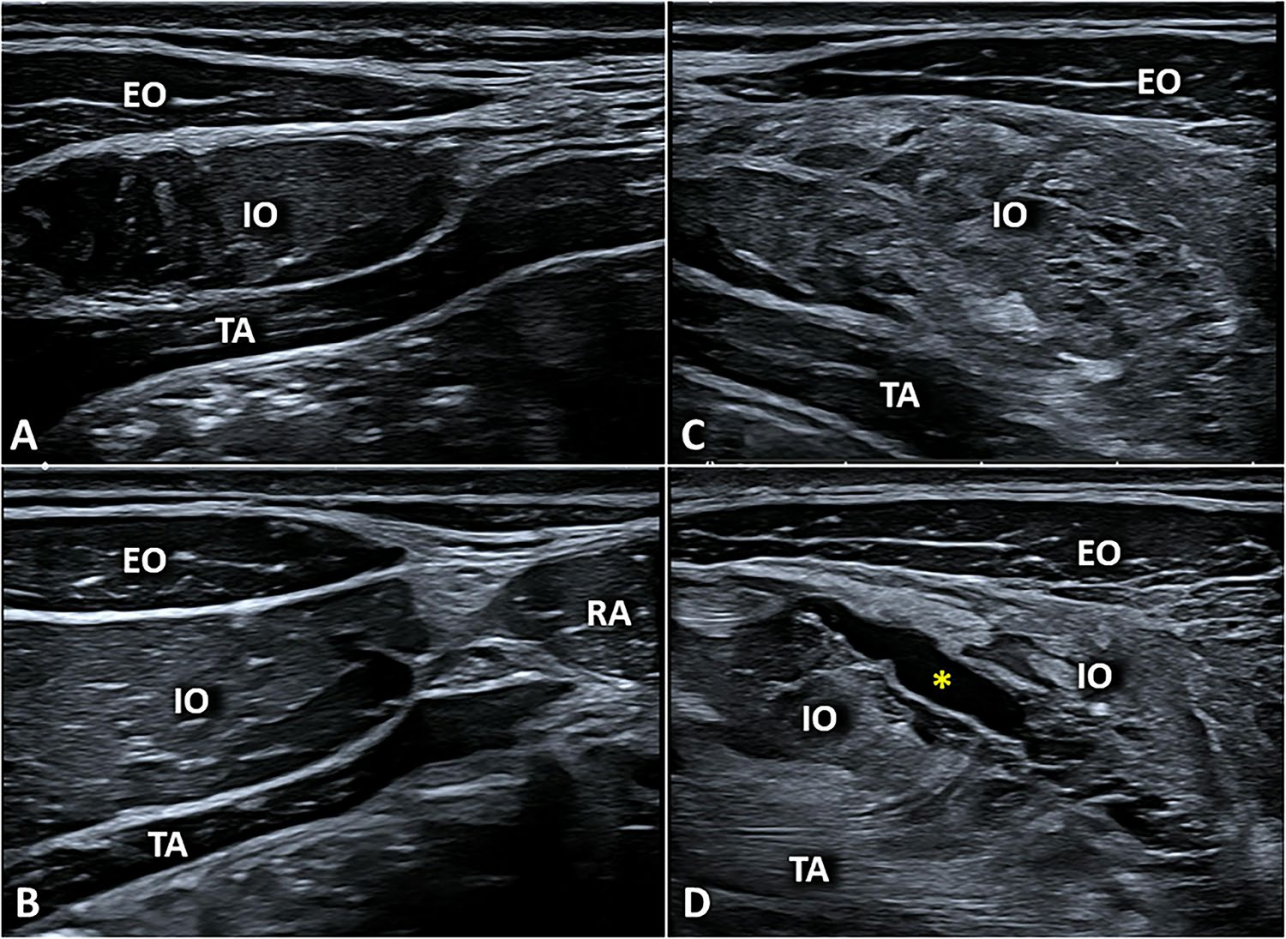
Comparative ultrasound scanning, in a transverse plane, of the normal (A, B) and painful (C) side of the anterolateral abdominal wall clearly shows a massive thickening and hyperechogenicity of the left internal oblique muscle *(IO)* due to severe intramuscular edema with a loss of the normal echotexture. Shifting the probe distally (D), disruption of its muscle fibers with a large intramuscular hematoma *(yellow asterisk)* has been identified confirming the post-traumatic myofascial injury with a sparing of the overlying external oblique muscle *(EO)*. *TA* transversus abdominis muscle, *RA* rectus abdominis muscle
